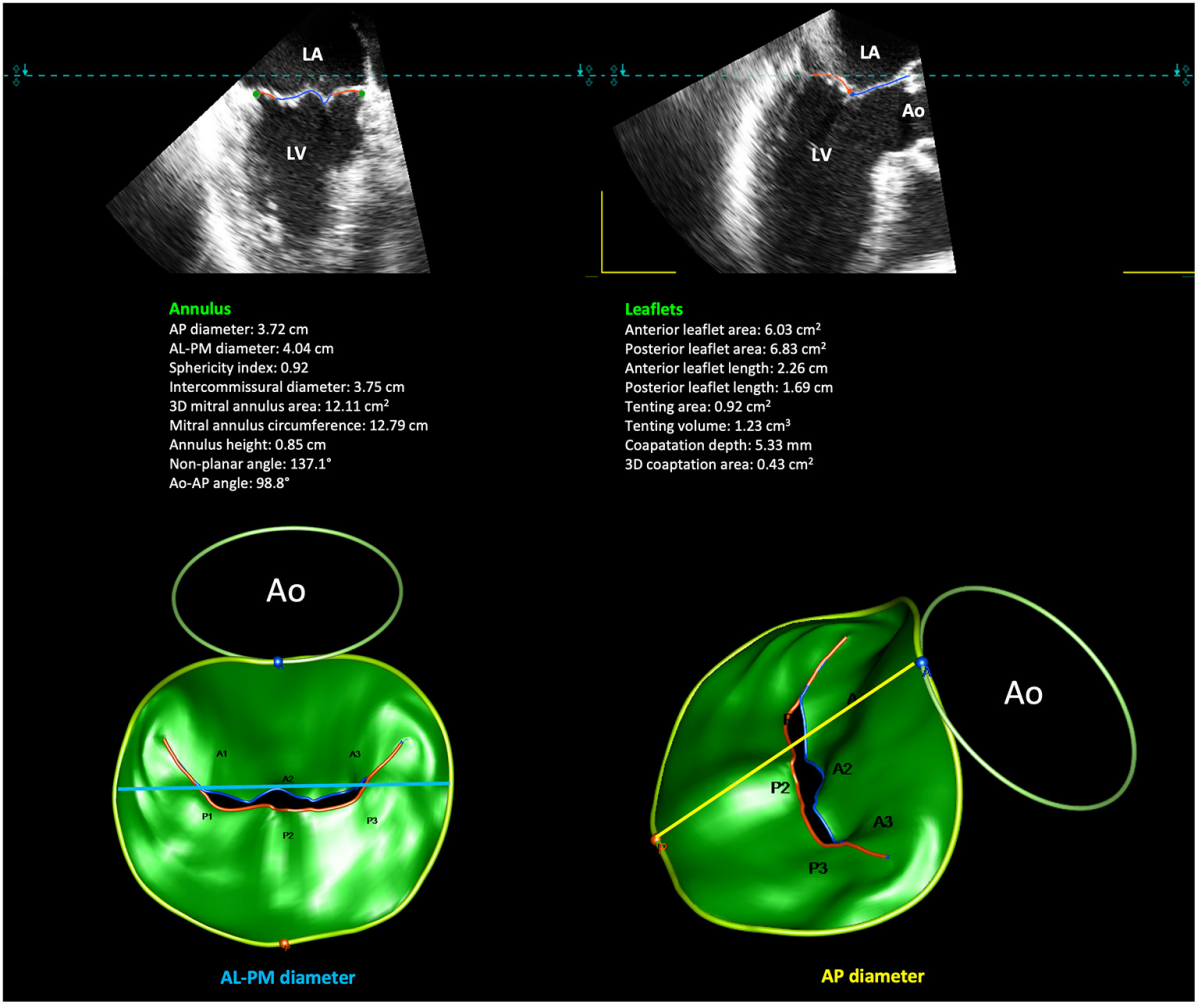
Quantitative modeling of the MV leaflets and annulus by 3DE. Shown is an example of 3D MA reconstruction and semi-automatic computation of 3D MA and leaflets measurements in a case of P2 prolapse. 3DE, three-dimensional echocardiography. MA, mitral annulus.
